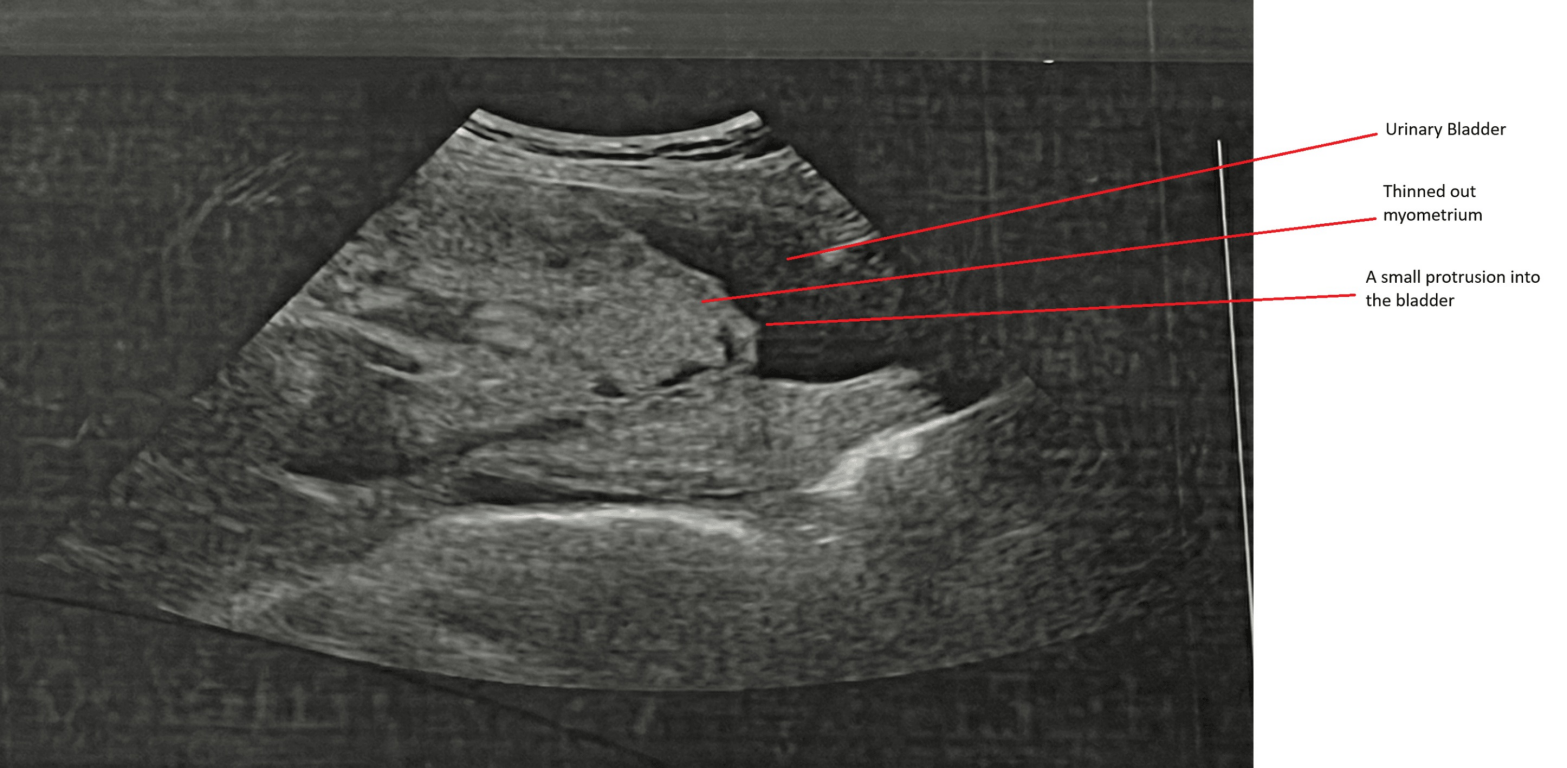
USG scan of the placenta. Obsteric USG demonstrating anterior placenta pervia with thinning of the myometrium and focal protrusion toward the urinary bladder (arrows), suggestive of placenta percreta. USG: ultrasonography.
